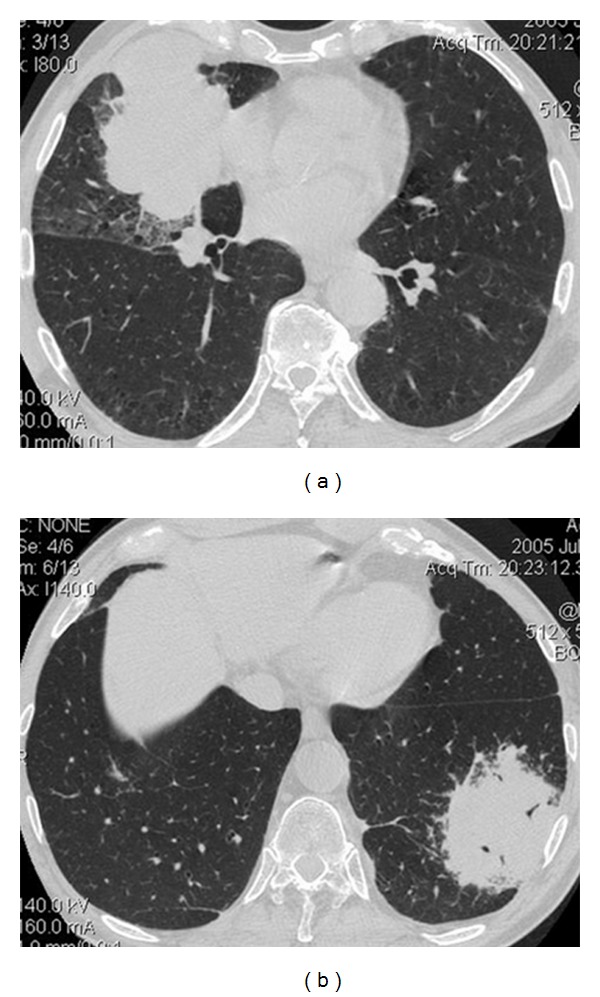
Chest CT on lung window settings shows a lobulated ill-defined mass surrounded by ground-glass opacity in the middle lobe (a) and an ill-defined masslike opacity with air bronchogram centrally in the left lower lobe (b).
